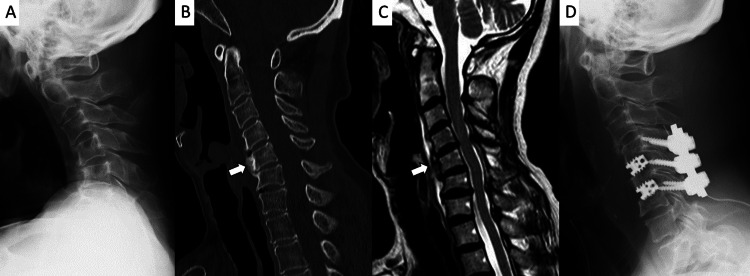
Preoperative Images A: Lateral plain radiograph showing cervical kyphosis; B: Cervical CT and C: MRI reveal local kyphosis at the C4–C6 levels with bony changes on the anterior vertebral bodies (arrows); D: Anteroposterior and posterior fixation was performed from C4 to C6.
